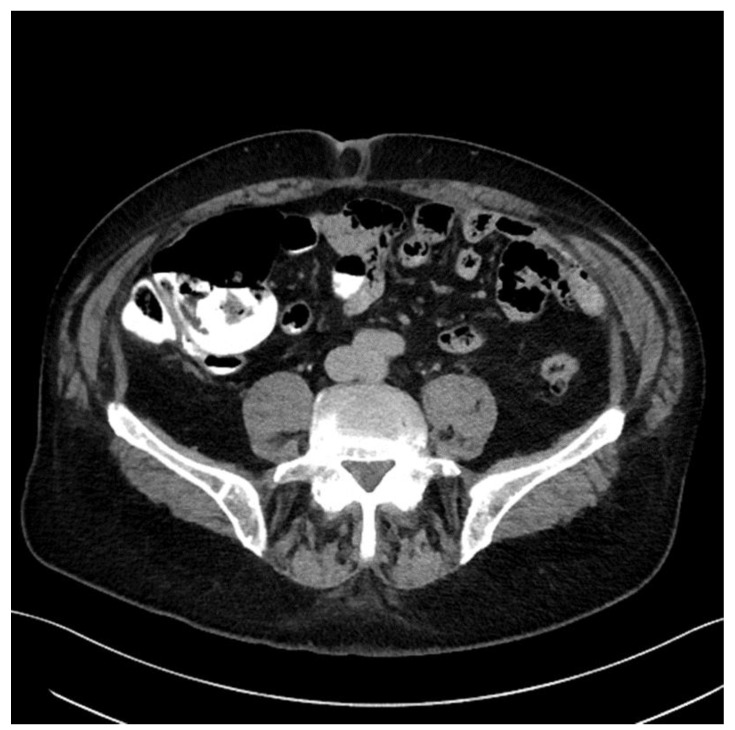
Computed tomography (September 2021).
